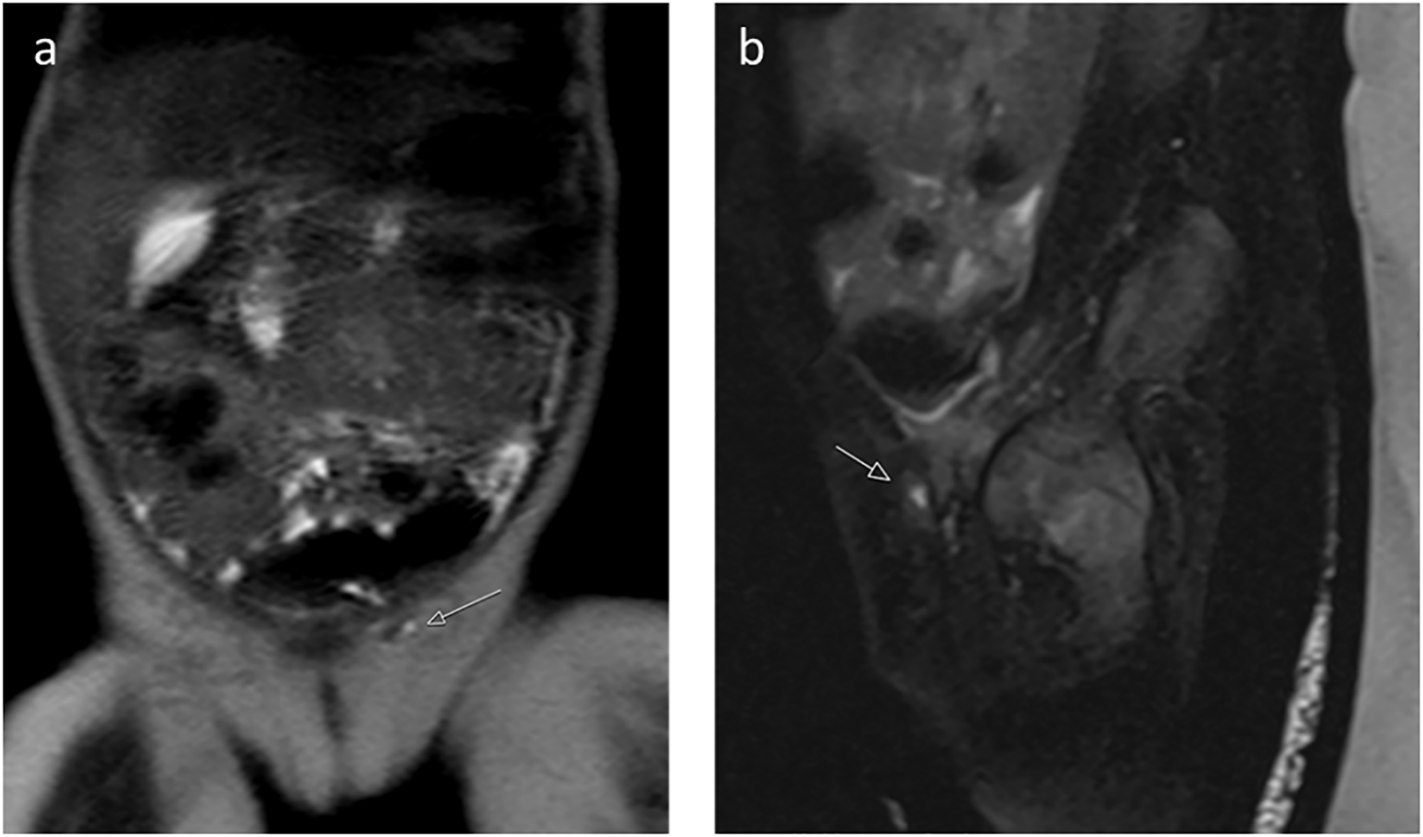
(a) Coronal T2-weighted image and (b) sagittal T2-weighted image with fat saturation reveal a small, high-signal intensity structure in the left inguinal canal, suggestive of undescended gonadal tissue, and showed a male-type urethra with a small vaginal-like structure connecting to the urogenital sinus (Patient 1).
